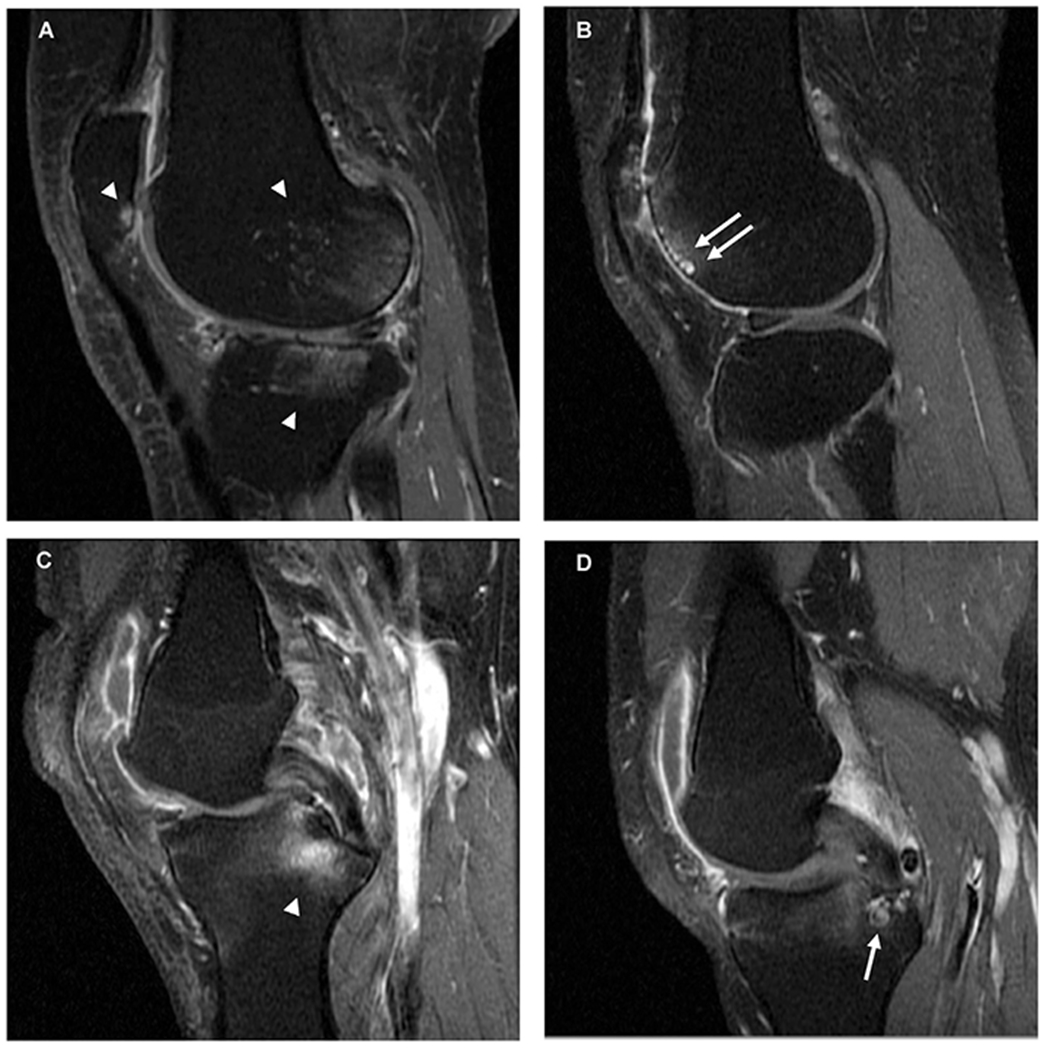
Bone marrow lesion (BML) types on sagittal T_1_-w fat suppressed contrast-enhanced MRI. (A) Subchondral BMLs at the posterior femoral condyle, posterior patella and lateral tibial plateau with ill-defined hyperintense (oedema-like) signal (white arrowheads). (B) Subchondral BML with cyst-like components (white arrows) in the anterior femoral condyle. (C) Ligament-based BML associated with the attachment of the posterior cruciate ligament (PCL) (odema-like signal extends into the subchondral bone) (white arrowhead). (D) Cyst-like component (white arrowhead) of a ligament-based BML in the tibia associated with the posterior cruciate ligament attachment.
